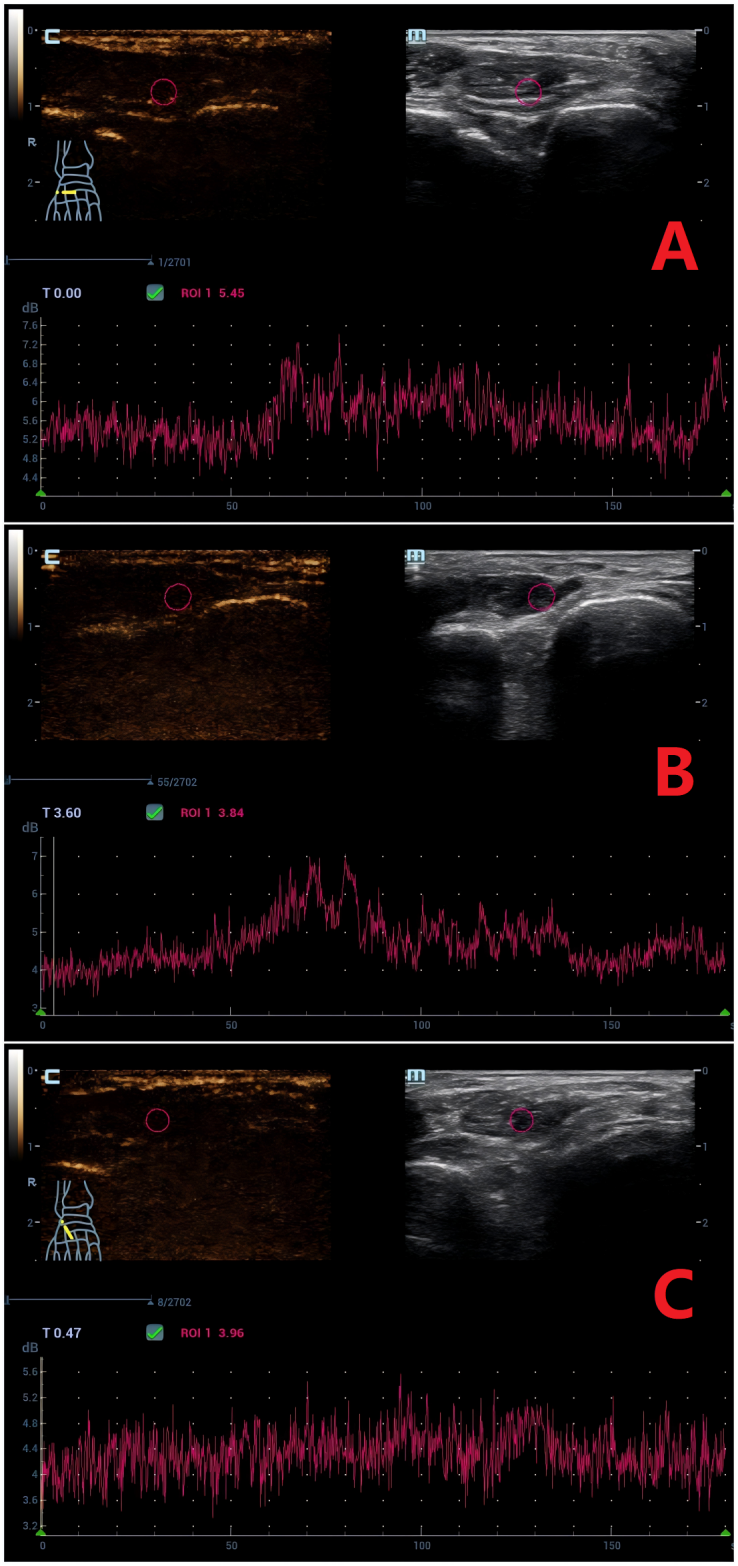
Time-Intensity Curves (TICs) of Contrast-Enhanced Ultrasound (CEUS) for the Extensor Hallucis Brevis Muscle. (A) Normal group: Arrival time (AT) = 50.5 s, peak intensity (PI) = 7.0 db. (B) DM group: AT = 51.6 s, PI = 6.7 db. (C) DM+MC group: AT = 89.5 s, PI = 5.0 db. CEUS parameters demonstrate delayed contrast arrival (AT) and reduced perfusion (PI) in the DM+MC group.
